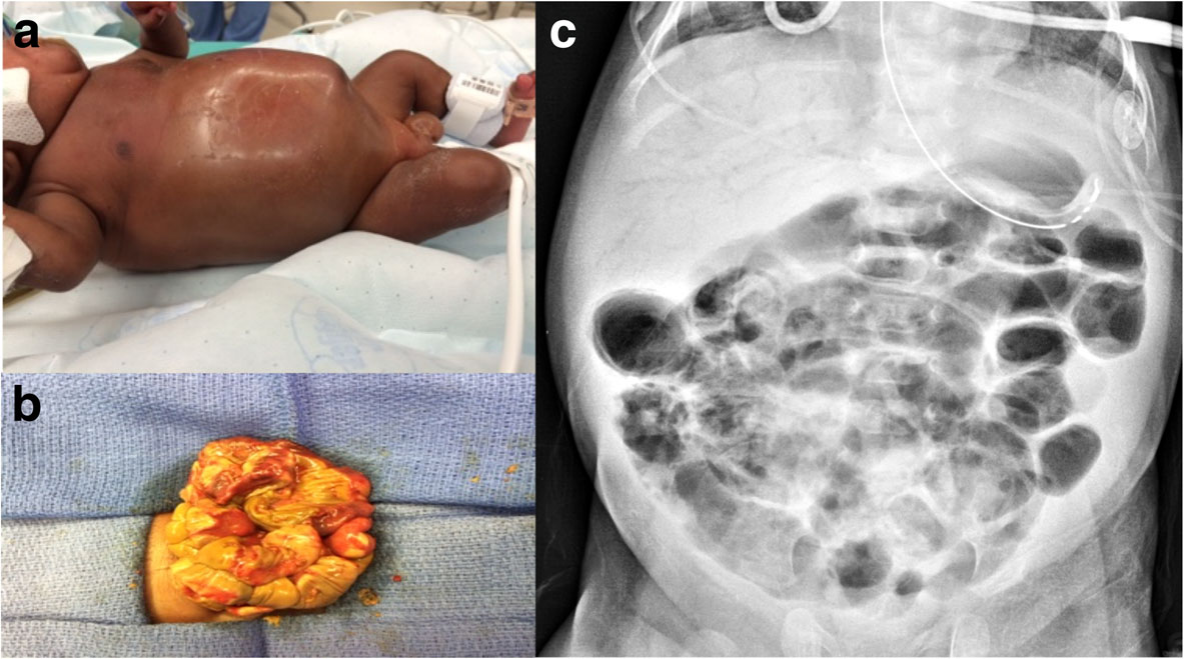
Clinical findings of Necrotizing Enterocolitis. a Abdominal distension and erythema frequently seen in an infant with necrotizing enterocolitis. b Necrotic bowel found upon surgical exploration for necrotizing enterocolitis. c Abdominal radiograph demonstrating portal venous gas and pneumatosis intestinalis
